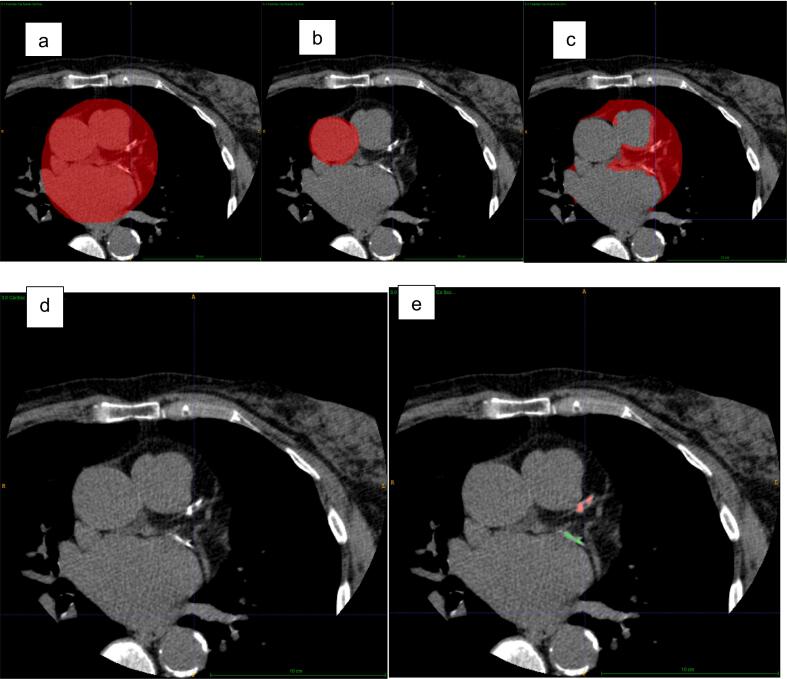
Examples of gated CT study showing Segmentation; a: Cardiac Segmentation; b: Aorta Segmentation; c: Epicardial Fat Segmentation; and d: Showing Coronary Artery Calcification; e: Model detected Coronary Artery Calcification and different colour in coronary artery territories. Red colour and green colour showing LAD and LCx respectively.
